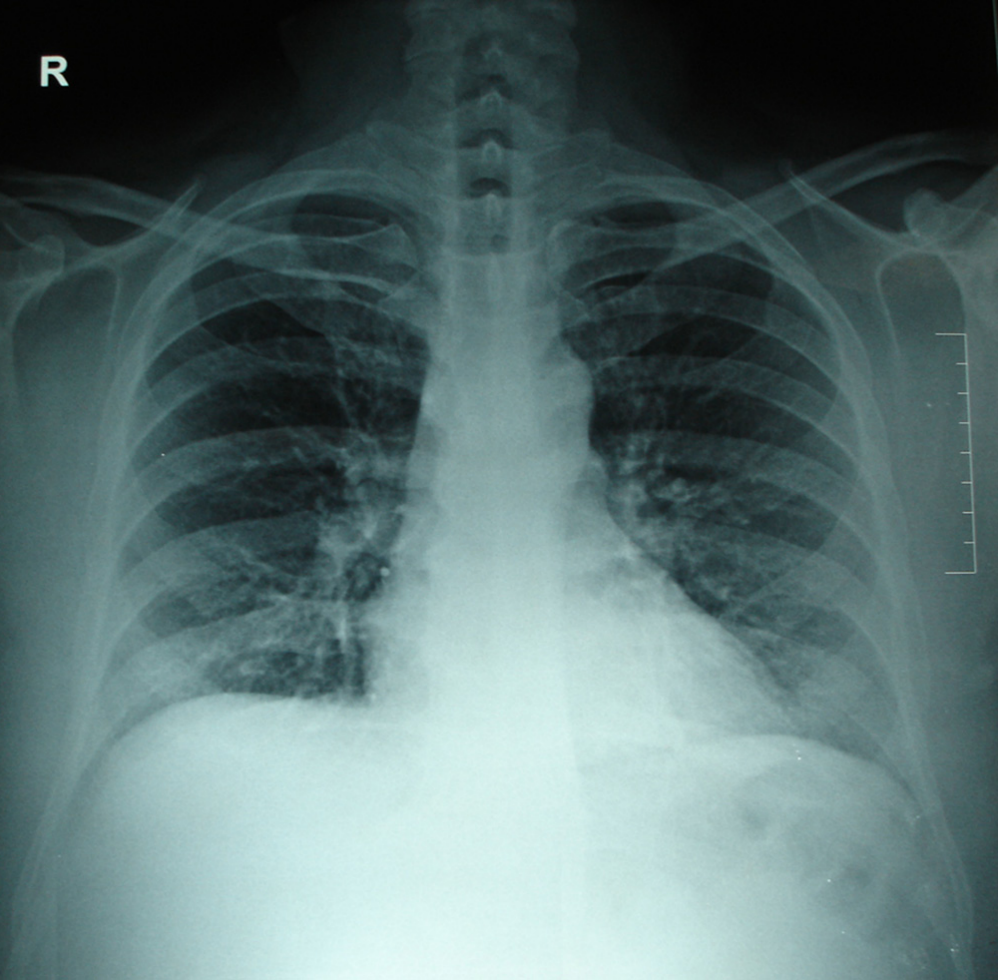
X-ray chest 6-weeks after ligation of the fistula. X-ray of the chest (PA-view), taken 6-weeks after ligation of the fistula, showing resolution of the pleural effusion with no recurrence.
